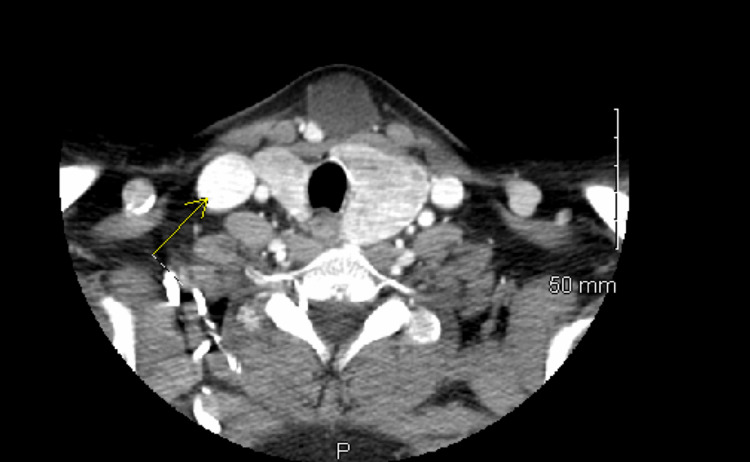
CT with contrast: a thin-walled cystic lesion was located near the thyroid gland.
